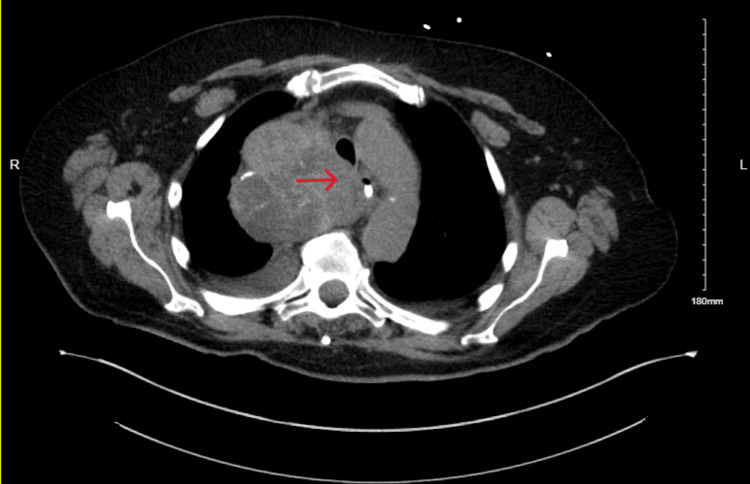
Mediastinal mass seen on the CT scan of the chest causing compression of the esophagus and trachea.
